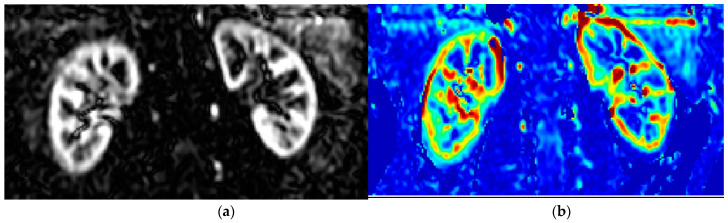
Representative perfusion weighted image (a) and the resulting RBF map (b) from the renal ASL (CASL) MRI technique on a healthy volunteer.
